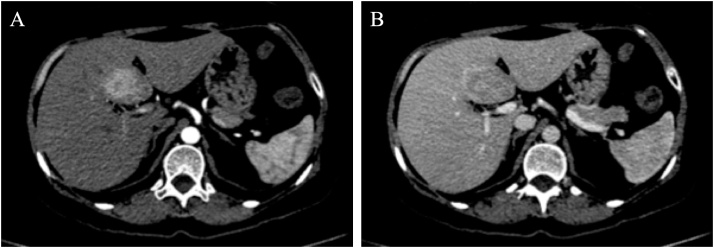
Preoperative CT scans: arterial phase (A); and portal phase (B).
